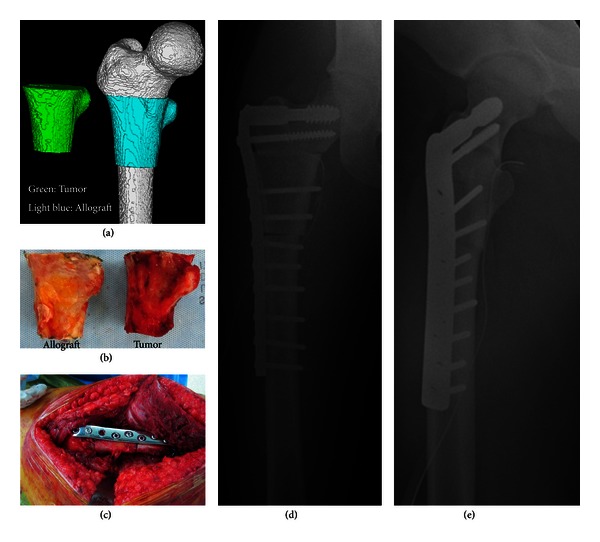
(a) Bone allograft was selected according to the shapes comparison between host and donor. The original tumor diagnosis was a chondrosarcoma. (b) Allograft was selected and tumor was resected. (c) Allograft was fixed in the patient through a plate and screws. (d) and (e) Postoperative X-rays.
